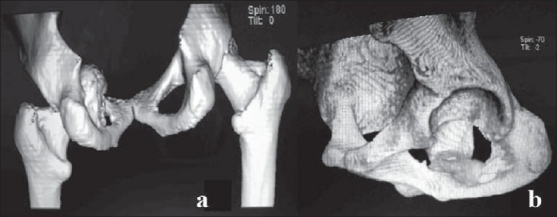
(a) 3D reconstructed CT, viewed from behind depicts the intrapelvic dislocation of left hip. (b) Subtracted 3D reconstructed CT image showing the integrity of the left acetabulum with a defect in the inferior aspect of medial wall
